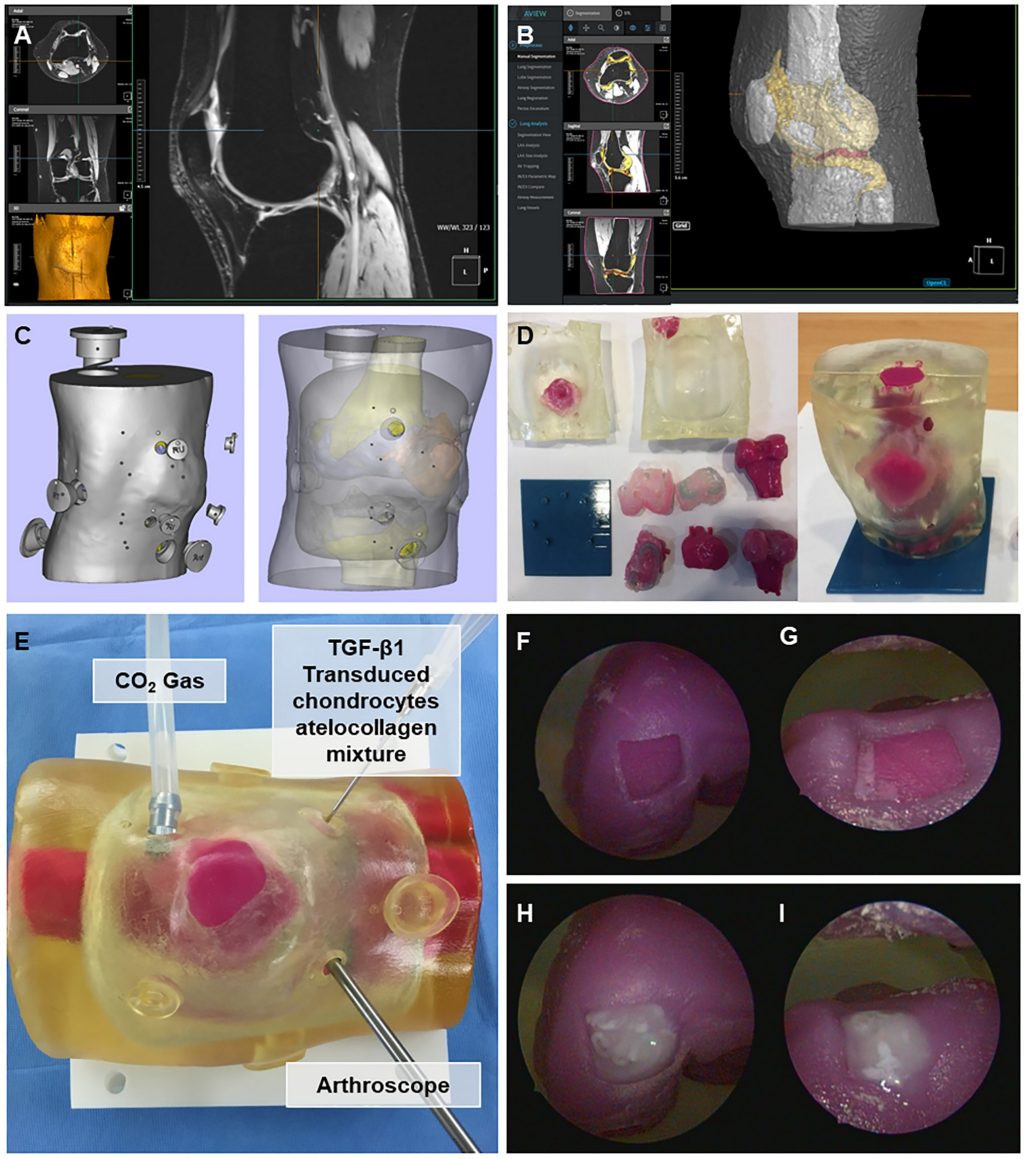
Process of knee joint model production using 3D printing technique. (A) Image segmentation based on the knee T2 magnetic resonance imaging data, (B) conversion of each divided part to stereolithography files, (C) implementation of functional elements with computer-aided design, and (D) output using a 3D printer. Arthroscopic application of TGF-β1-transduced chondrocyte-atelocollagen mixture. (E) Knee joint model from the 3D printing technique. Arthroscopic findings after applying the TGF-β1-transduced chondrocyte-atelocollagen mixture: (F) Medial femoral condyle defect, (G) trochlear defect, (H) application of the mixture to the medial femoral condyle defect, and (I) application to the trochlear defect.
